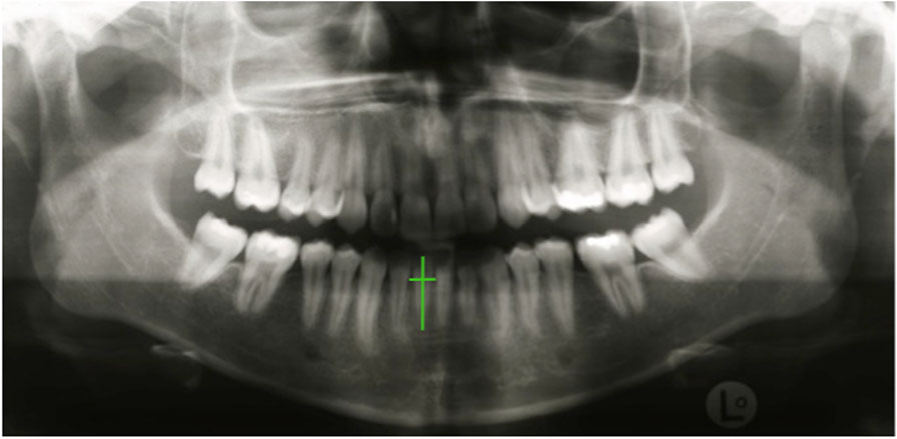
Orthopantomogram of a patient before orthodontic treatment. Lengths of the crown and root of the incisor with the torque discrepancy was measured and compared to the lengths of the crown and the root after treatment
